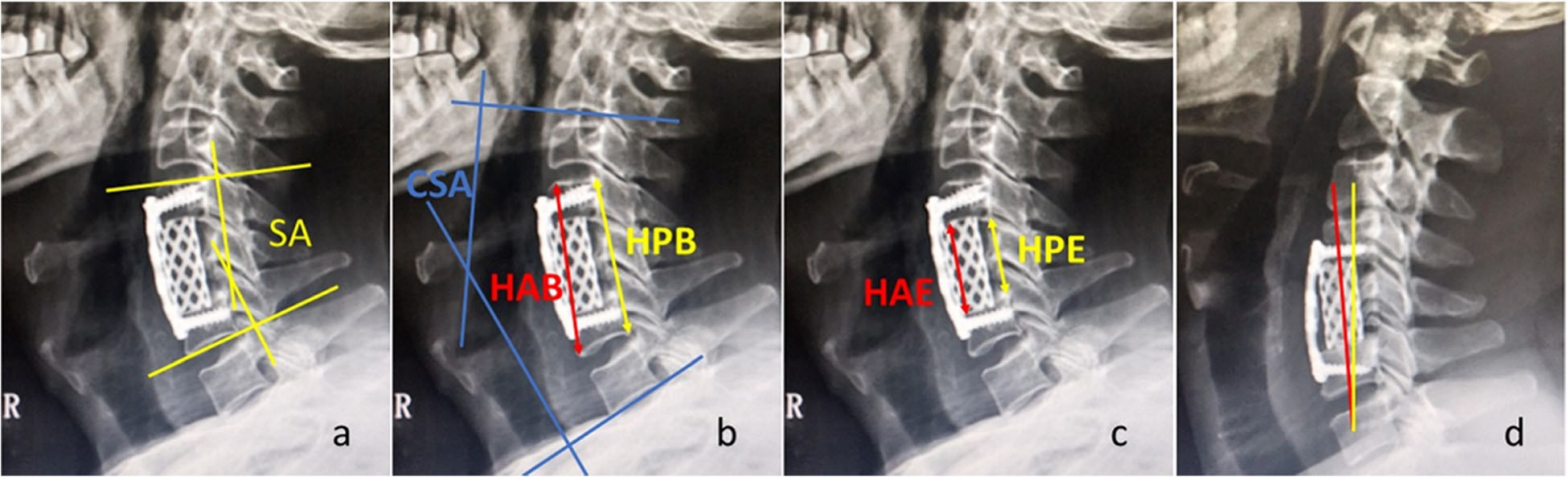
Sagittal radiograph showing a the segmental angle (SA) between the borders of endplates above and below the affected segment. b the cervical sagittal angle (CSA) formed between the lower endplate of C2 and C7. The height of anterior (HAB) and posterior border (HPB) were measured as the distance between the anterior and posterior points of the upper endplate of the superior vertebra and the lower endplate of the inferior vertebra. c The height of anterior (HAE) and posterior endplate (HPE) were measured as the distance between the anterior and posterior points of the lower endplate of the superior vertebra and the upper endplate of the inferior vertebra. d The alignment of TMC was defined as the angle formed between the central axis of TMC and the line through the midpoints of both endplates of adjacent vertebrae
